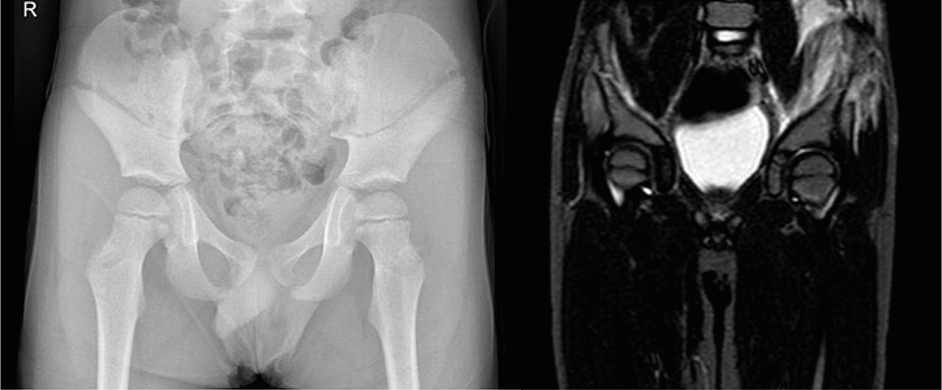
Symmetric bilateral iliac wing fracture with bone and intramuscular edema on additional MRI.
